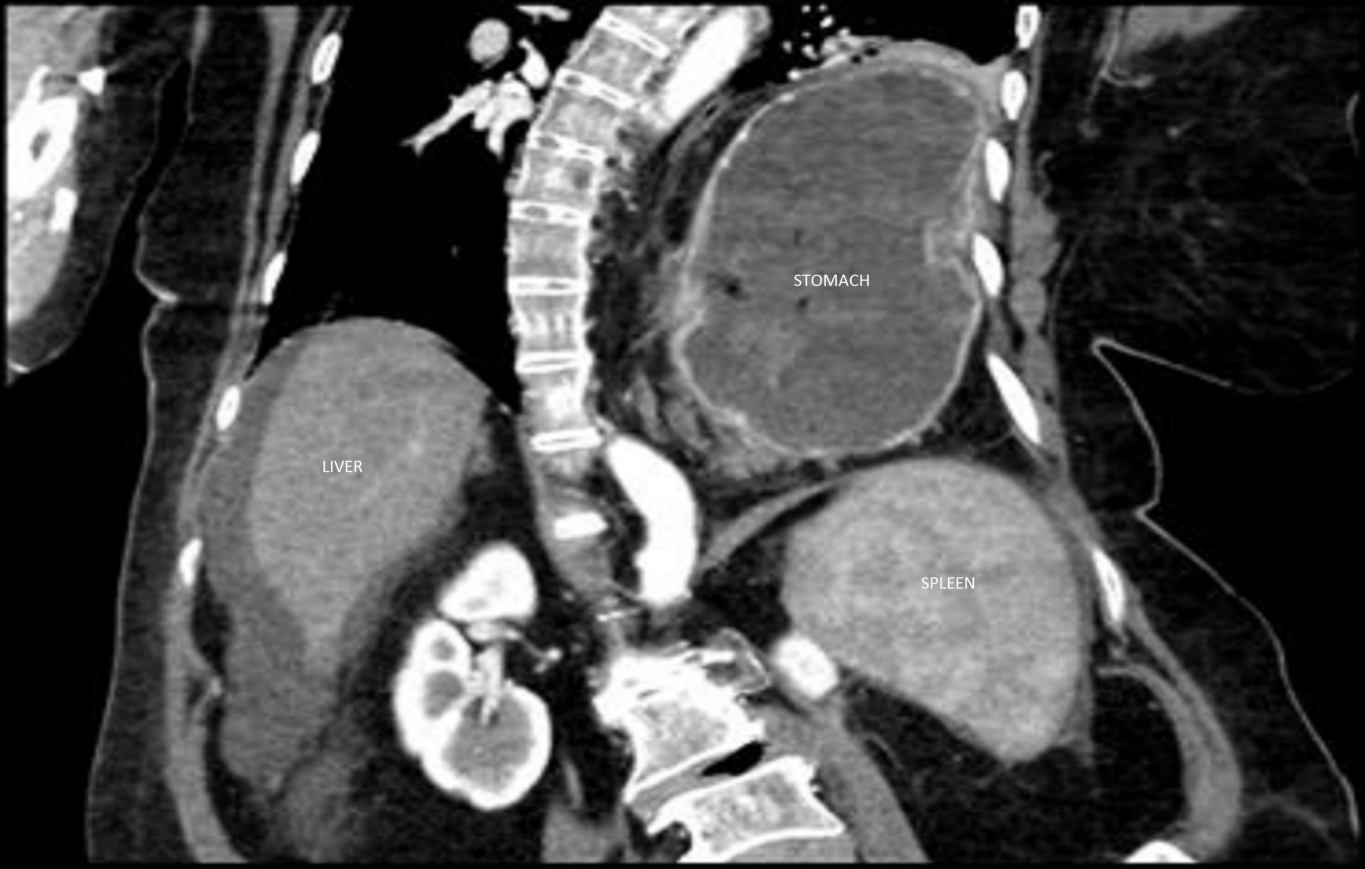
Contrast abdominal computed tomography (CT) scan. Coronal view showing an elevated left hemidiaphragm and a large hiatal hernia containing the stomach. The osseous structures demonstrate severe lumbar levoscoliosis and degenerative changes of the spine. Moderate ascites and splenomegaly were also noted.
